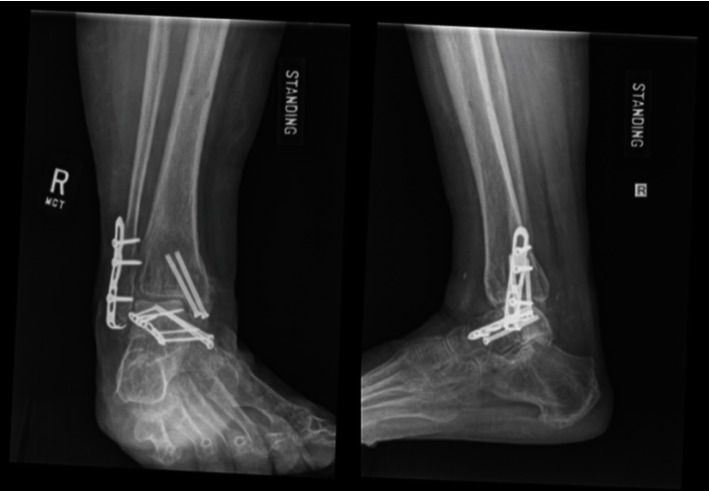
1-year follow-up; radiographs demonstrated union of fractures, early subtalar and tibiotalar arthritis, and improvement of prior sclerosis of talus without signs of collapse of talar dome.
